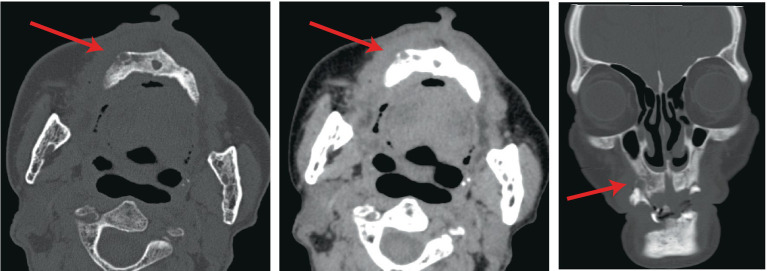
A plain computed tomography scan of the maxillofacial region indicated local bone destruction with surrounding soft tissue swelling on the right upper palate.
